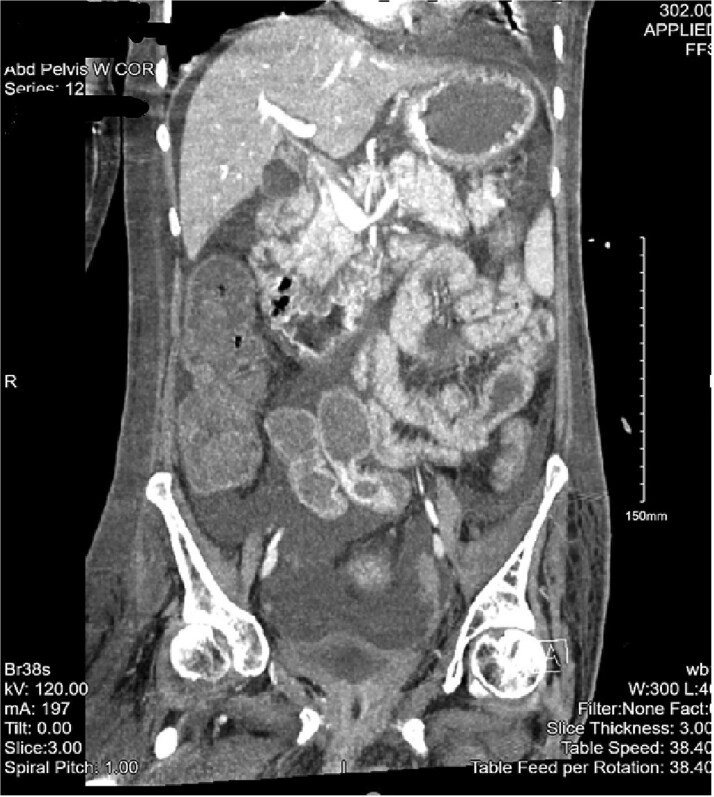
CT of the abdomen and pelvis with a coronal cross-section. Asymmetric wall thickening is observed in the cecum and ascending colon with adjacent fat stranding. Proximal dilation of small bowel loops with mesenteric stranding is also seen. Significant abdominopelvic free fluid is demonstrated. The CT findings correlate with mass versus inflammatory colitis. The read was addended to reflect concerns for cecal mass.
